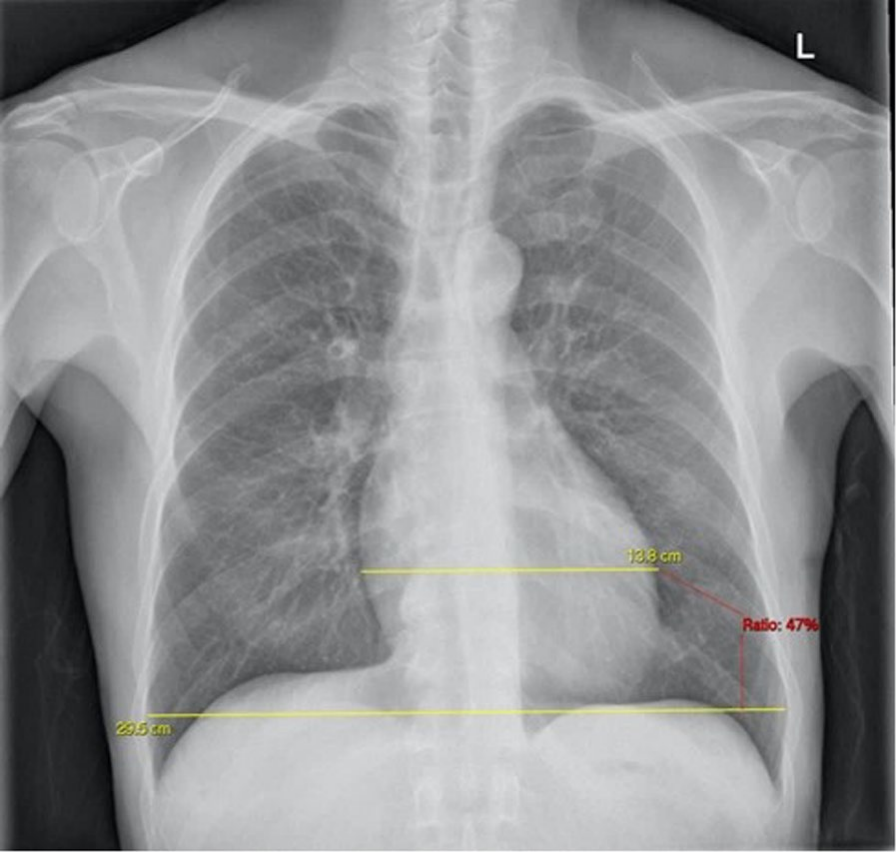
The cardiothoracic ratio on PA chest radiograph. The maximum transverse cardiac diameter is divided by the maximum transverse diameter of the thorax and multiplied by 100
